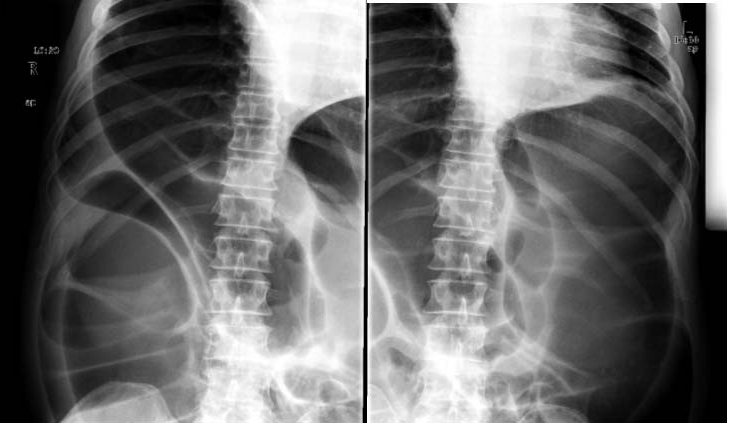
Radiographs demonstrating massively dilated large bowel. The transverse colon had a maximum diameter of 18 cm.
